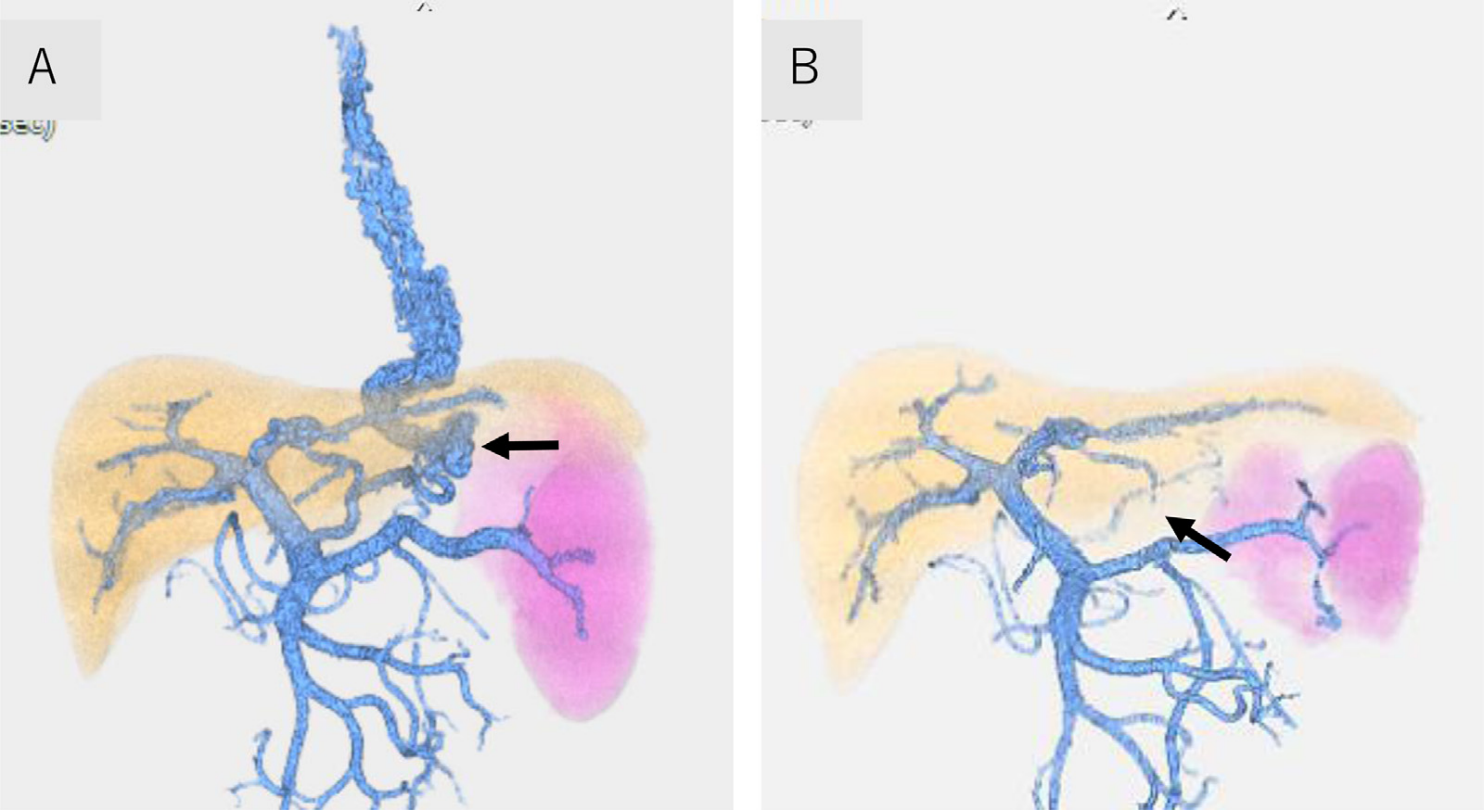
3D-CT reconstruction portal image before and after stepwise PSE and EISML. (A) 3D-CT reconstruction portal image before stepwise PSE and EISML shows the left gastric vein, gastric (arrow), and esophageal varices. (B) 3D-CT reconstruction portal image after stepwise PSE and EISML shows the disappearance of blood flow in esophagogastric varices. Partial thrombosis is observed in the left gastric vein (arrow). EISML, endoscopic injection sclerotherapy with multiple ligations; PSE, partial splenic embolization.
